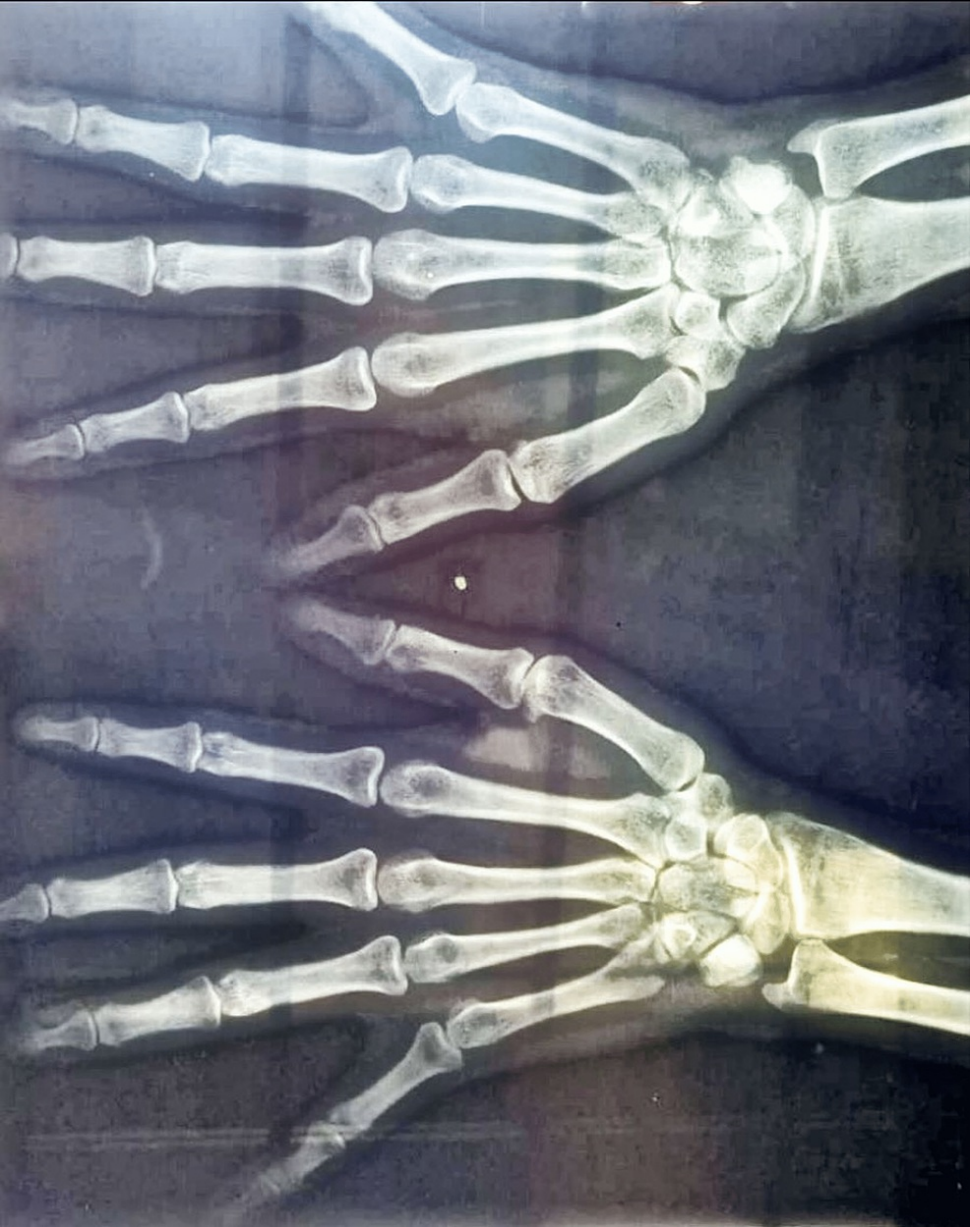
Posteroanterior X-ray of the right hand showing soft tissue swelling and no underlying bony involvement
